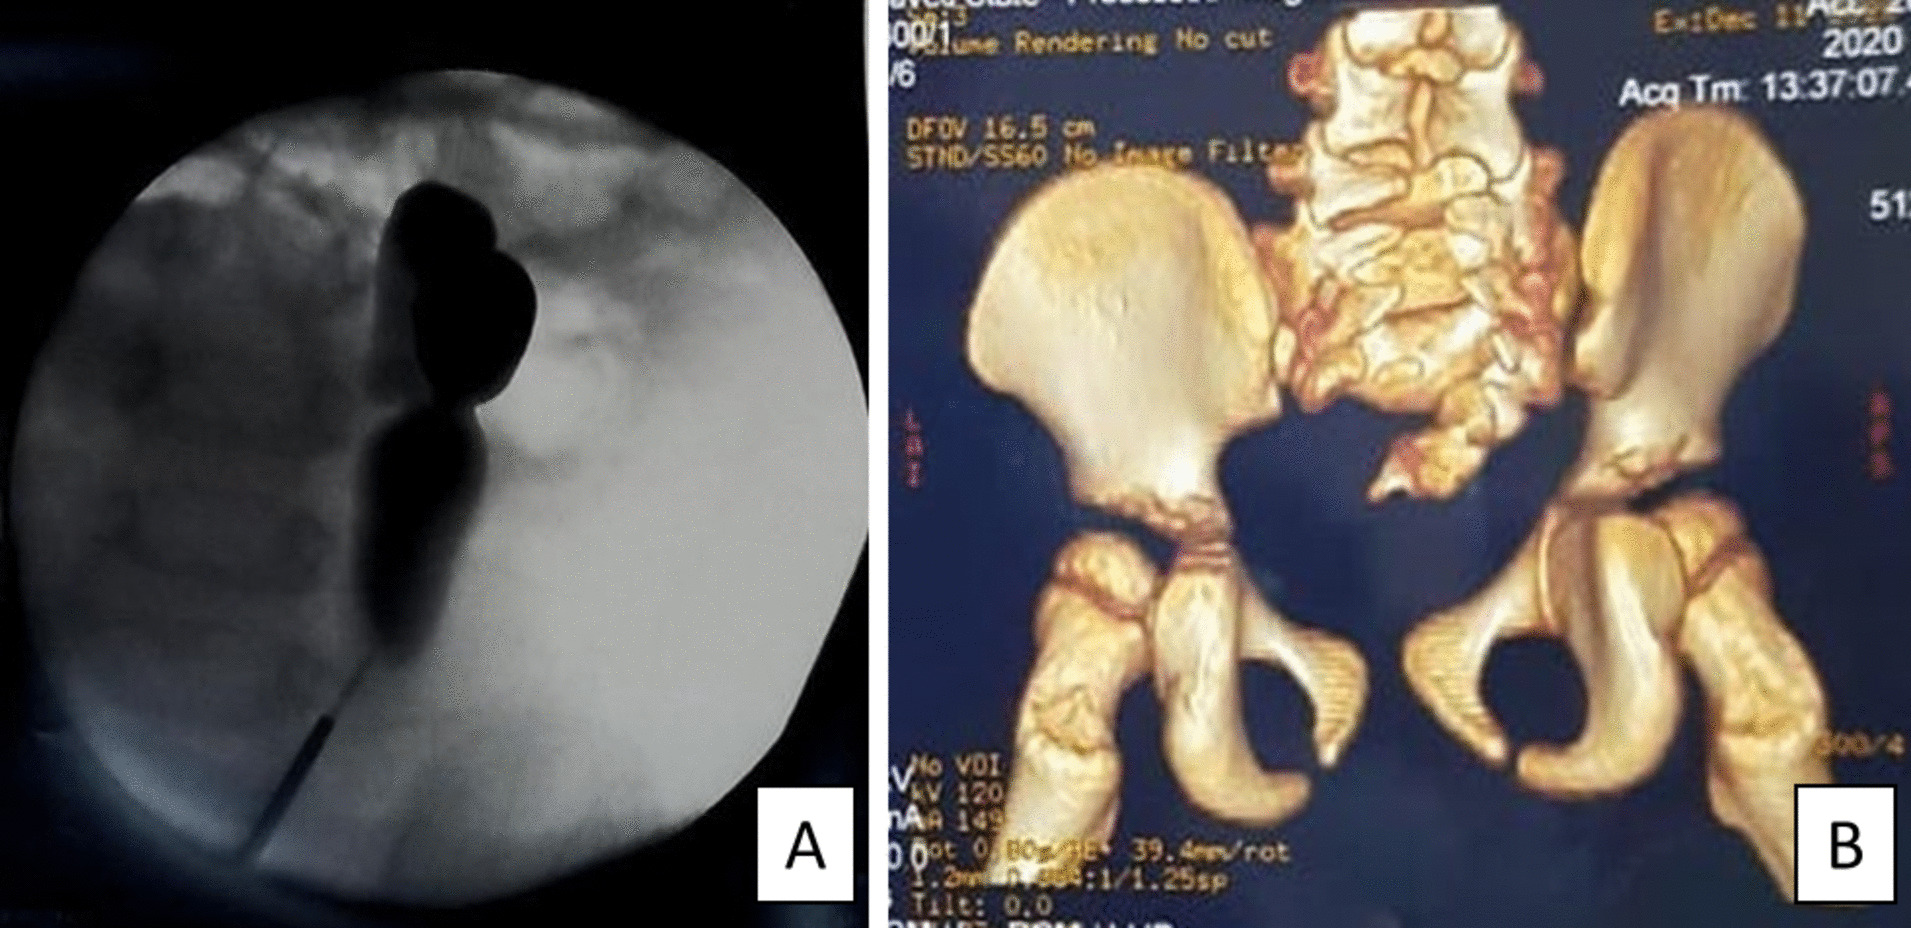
Radiological examination. A Retrograde Pyelography showed duplication of the left ureter and the absence of left collecting system. B MRI result showed bone defect at the posterior arch to confirm spina bifida
